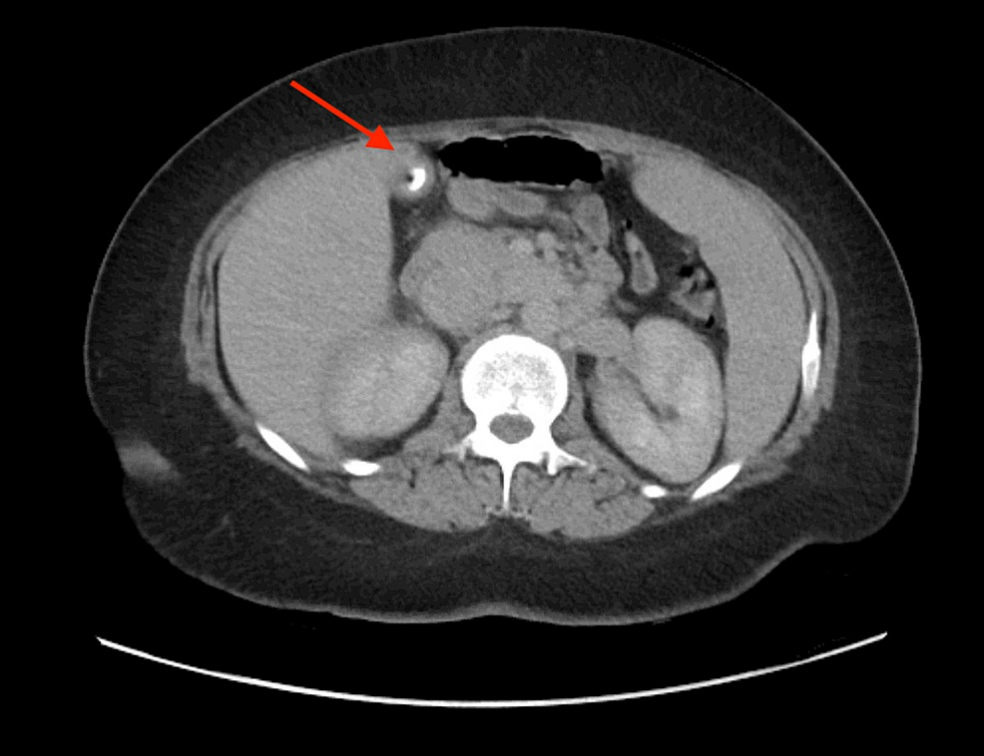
CT abdomen and pelvis CT, computed tomography. Arrow delineates calcified gallstones without obstruction.
